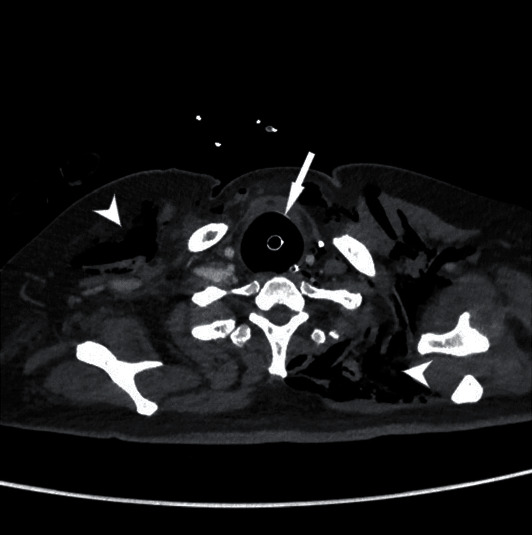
Neck CT. An overinflated tracheal tube cuff and dilated trachea can be seen compressing the cervical esophagus.
